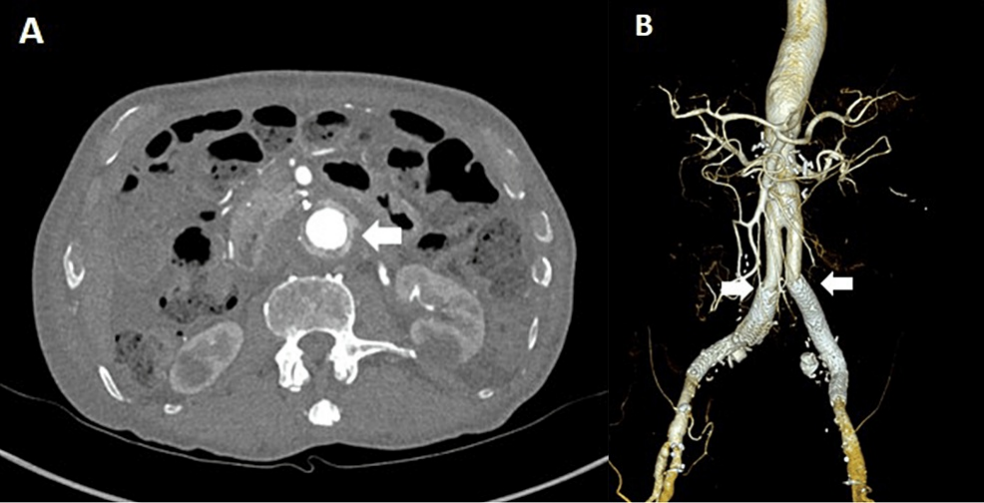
CTA on postoperative day 42 A: Axial cut at the level of proximal infrarenal anastomosis depicting felt strip at the anastomosis (white arrow); B: Three-dimensional reconstruction of the EVAR explantation depicting the distal anastomosis to the bilateral iliac limbs (white arrows) CTA: Computed tomography angiography, EVAR: Endovascular aortic aneurysm repair
